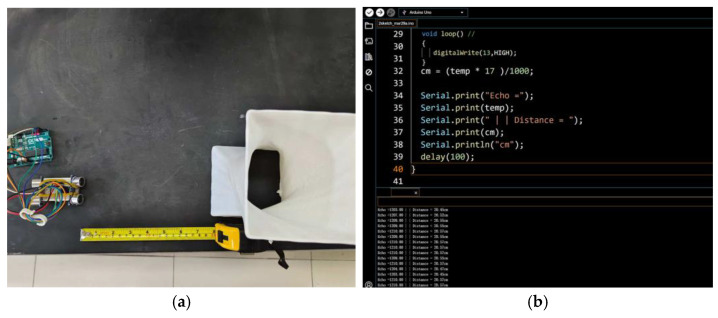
The actual distance measured by ultrasonic ranging. (a) Experiments utilize HC-SR04; (b) data from the ultrasonic sensor read via the PCB serial port.
